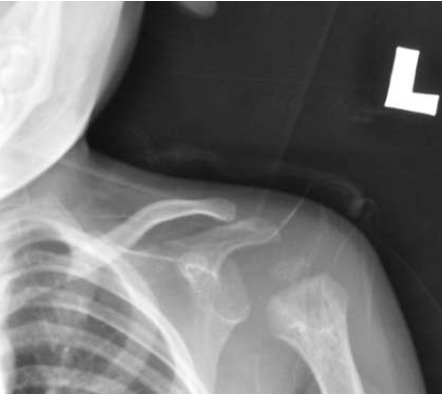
Fig. 2: Radiograph of left shoulder at 2 weeks of admission demonstrates lytic and sclerotic changes of the left proximal humerus consistent with osteomyelitic changes. F2:
